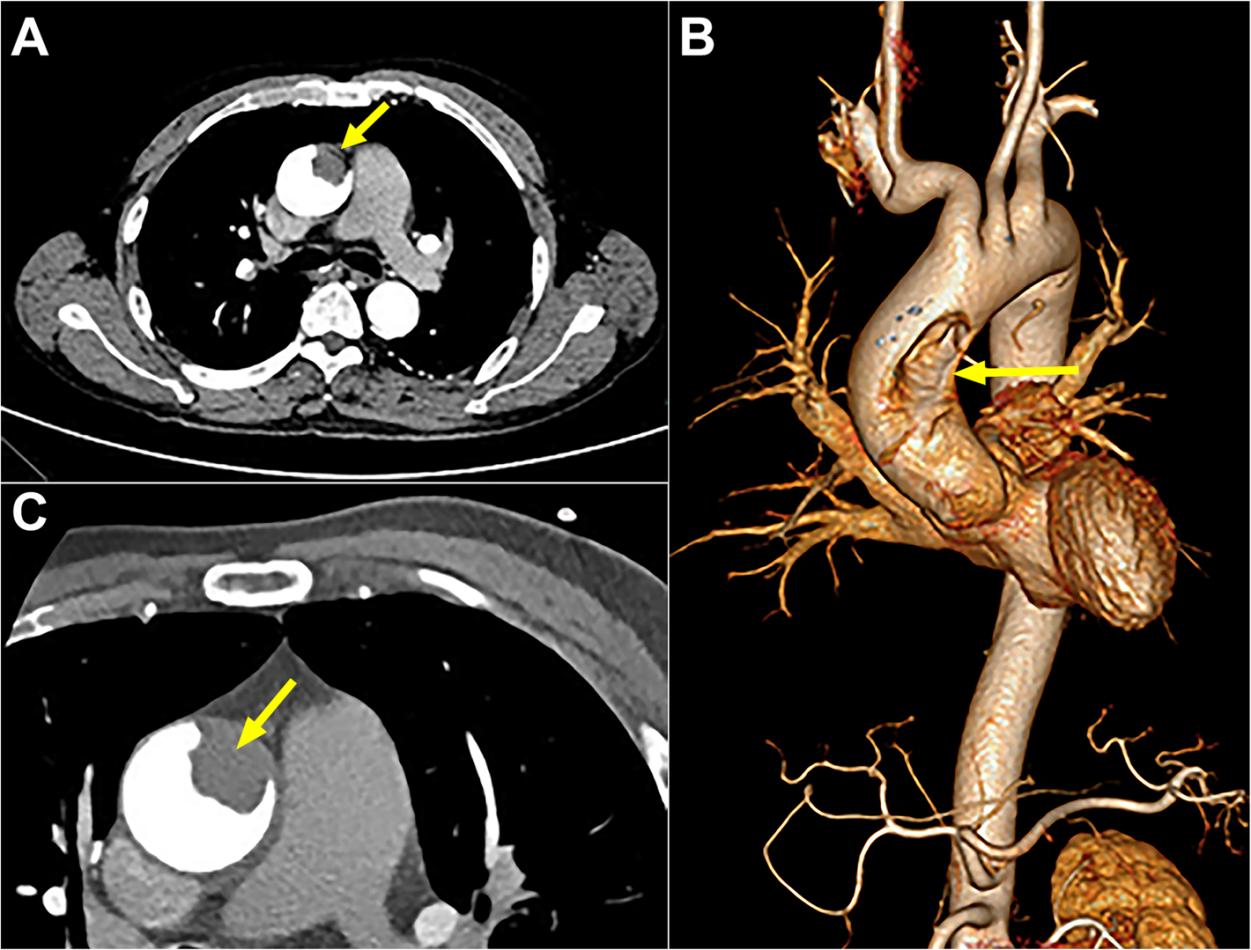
Preoperative imaging. A Thoracic contrast-enhanced computed tomography showed a low-density defect(arrow) with no enhancement in the left anterior wall of ascending aorta; B The 3-dimensional reconstruction displayed a long oval mass with broad basement(arrow); C Coronary computed tomography angiography showed that there was no occlusion of coronary ostia and confirmed a sessile mass with no mobility(arrow)
